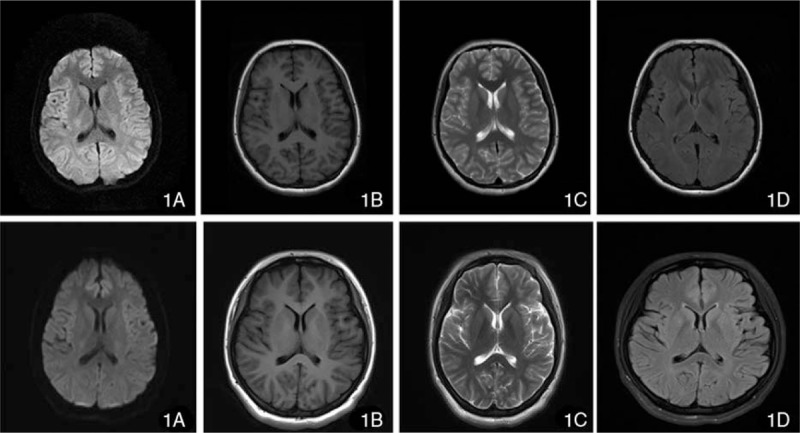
(1A–1D) Craniocerebral MRI scanning of patient 1. DWI, T1, T2, and Flare images showed no abnormalities. (2A–2D) Craniocerebral MRI scanning of patient 2. DWI, T1, T2, and Flare images showed no abnormalities.
